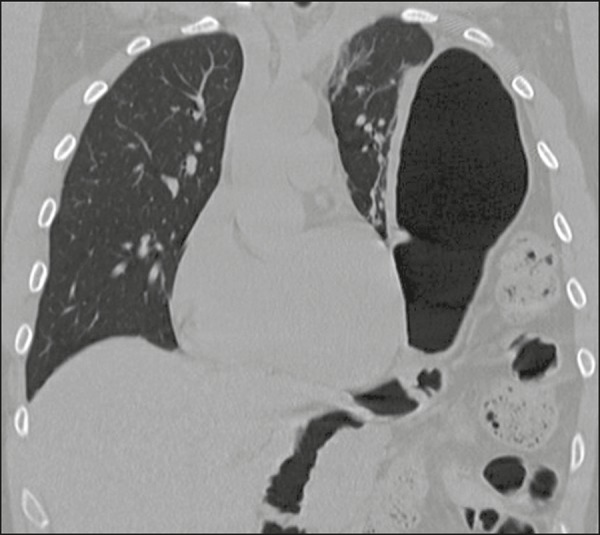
Coronal CT reconstruction of the thorax showing traumatic
